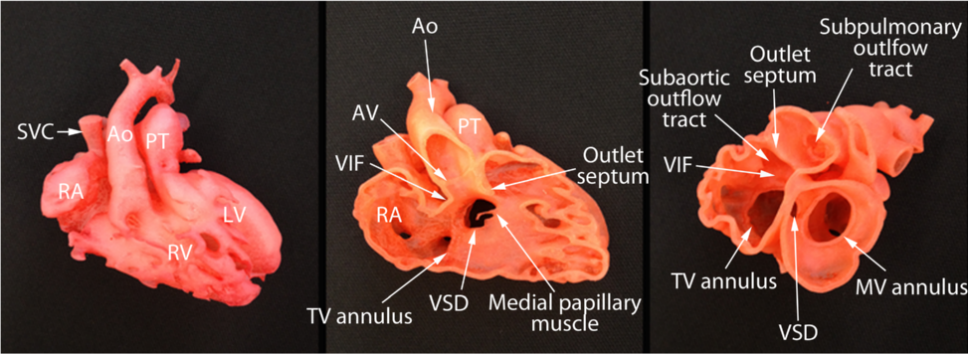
Photographs of the 3D print models of a case with double outlet right ventricle. Although echocardiograms showed that the ventricular septal defect (VSD) is remote from both arterial valves, 3D models show that the VSD is able to be routed to the aortic valve allowing biventricular repair. Ao, aorta; AV, aortic valve; LA, left atrium; LV, left ventricle; MV, mitral valve; SVC, superior vena cava; TV, tricuspid valve; VIF, ventriculoinfundibular fold
